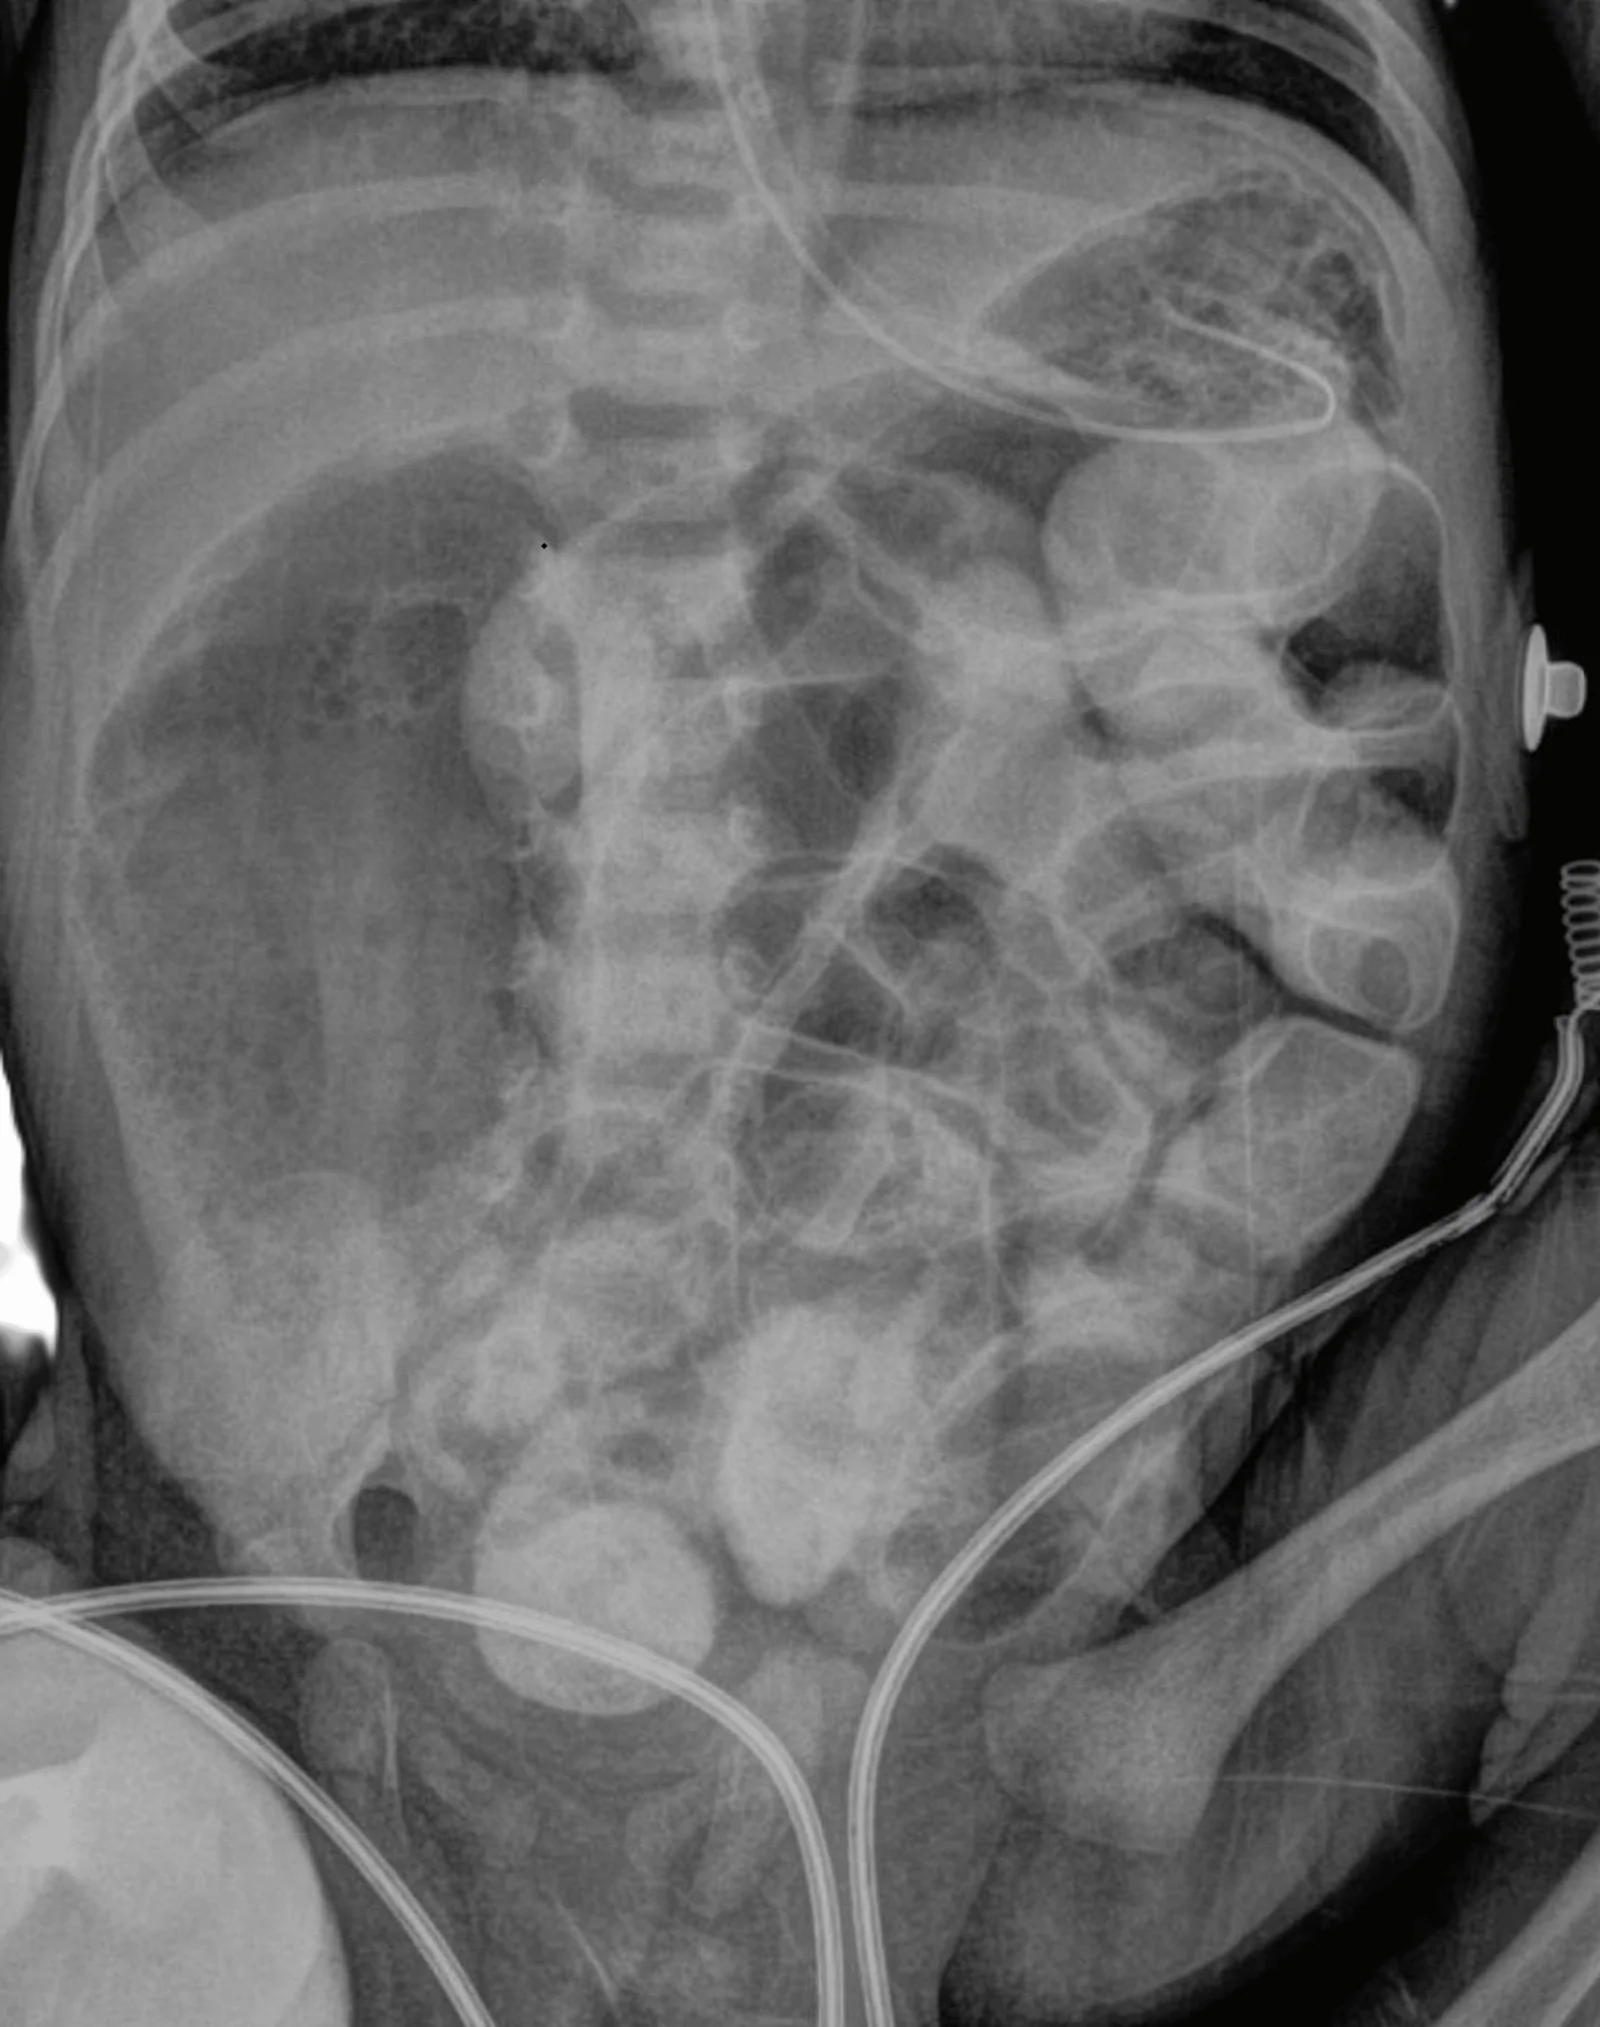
Preoperative oral contrast study Abdominal radiograph obtained after administration of oral contrast through an orogastric tube, showing passage of contrast into the small bowel without opacification of the colon. The tip of the orogastric tube projects over the stomach.
